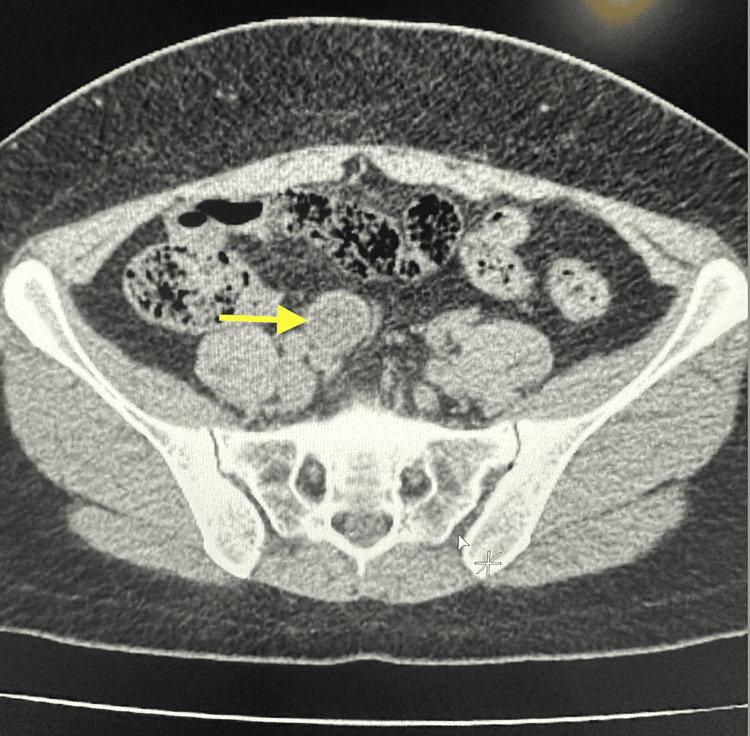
In this axial view CT scan of the abdomen, the yellow arrow is pointing to a 2.3 x 2.4 x 5.2 cm well-circumscribed cystic tubular mass contiguous with the base of the cecum with curvilinear mural calcification suspicious for AMN. CT: computed tomography; AMN: appendiceal mucinous neoplasm
